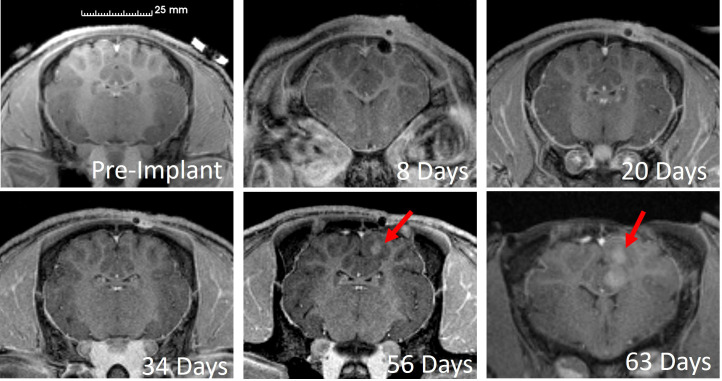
Longitudinal CE-MRI for a representative Oncopig. Tumor, depicted by the red arrow, was first observed during the imaging session 56 days after inoculation.
